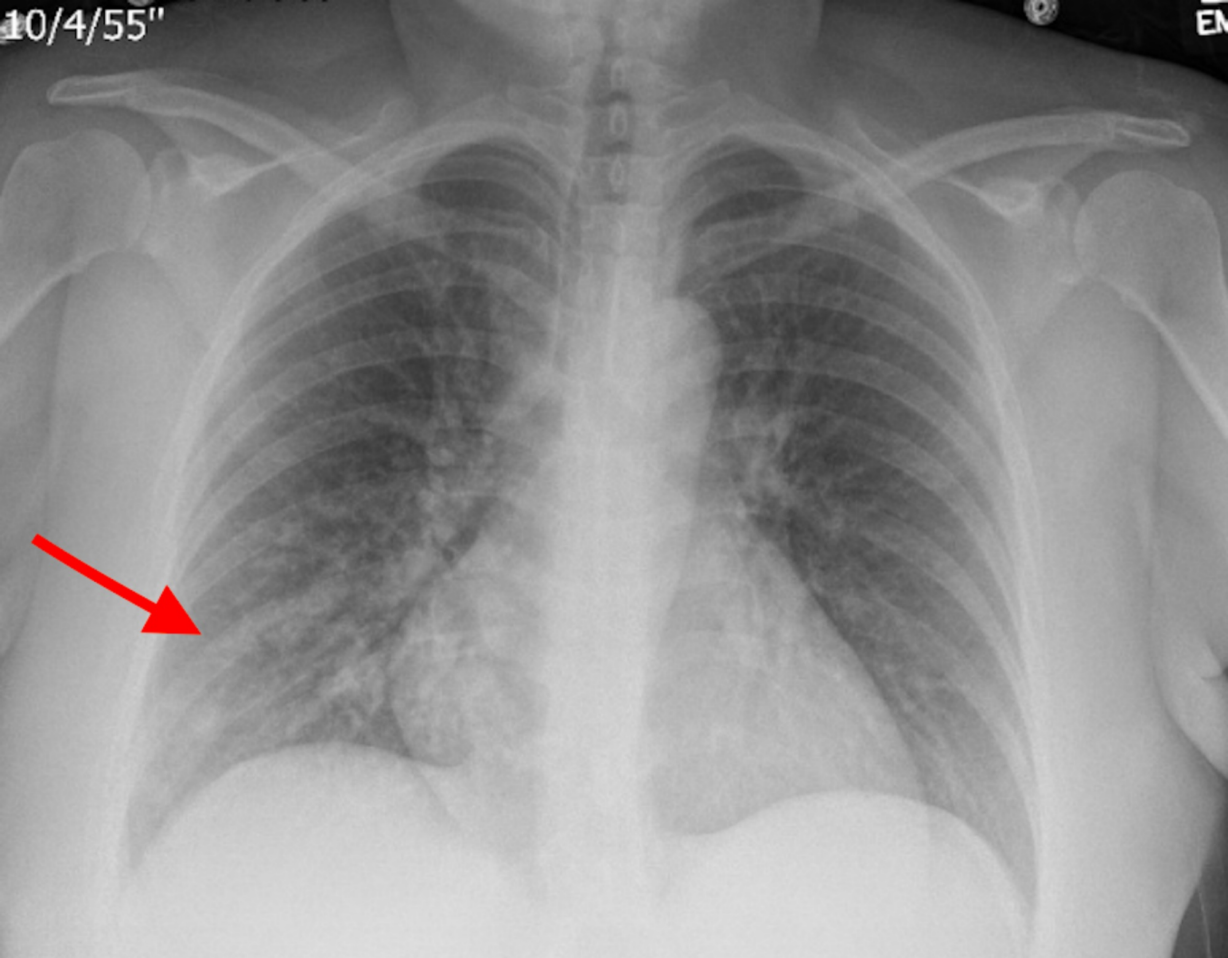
Chest X-ray showing patchy infiltrate in the right lower lung base (red arrow).
